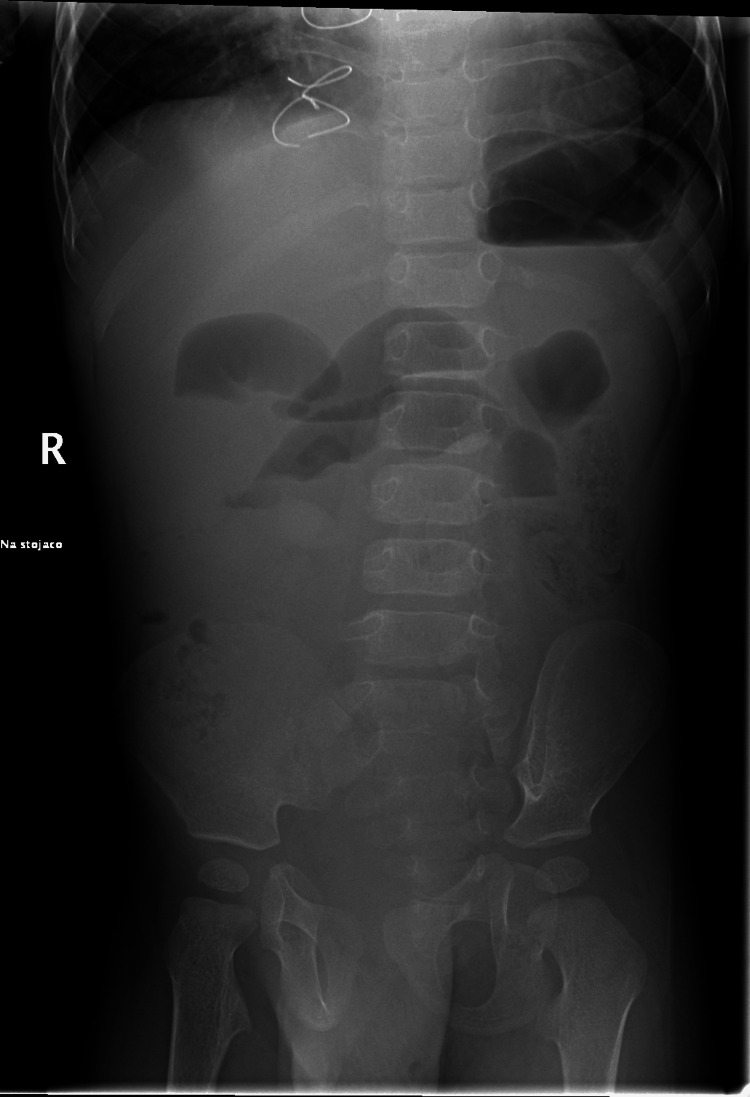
Abdominal X-ray showing dilated loops with fluid levels confirming intestinal obstruction
